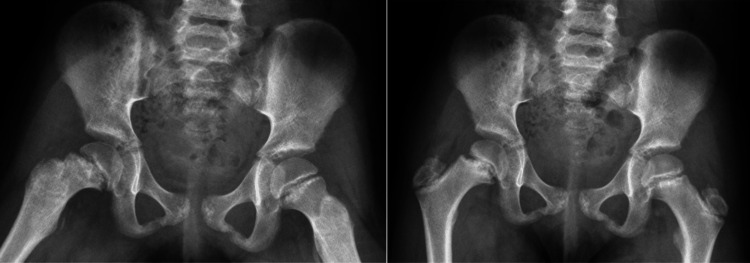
Anterioposterior and frog leg X-rays show a chronic, stable right SCFE with widening left physis in an overall skeletally immature female patient (open triradiate cartilages). SCFE: slipped capital femoral epiphysis
